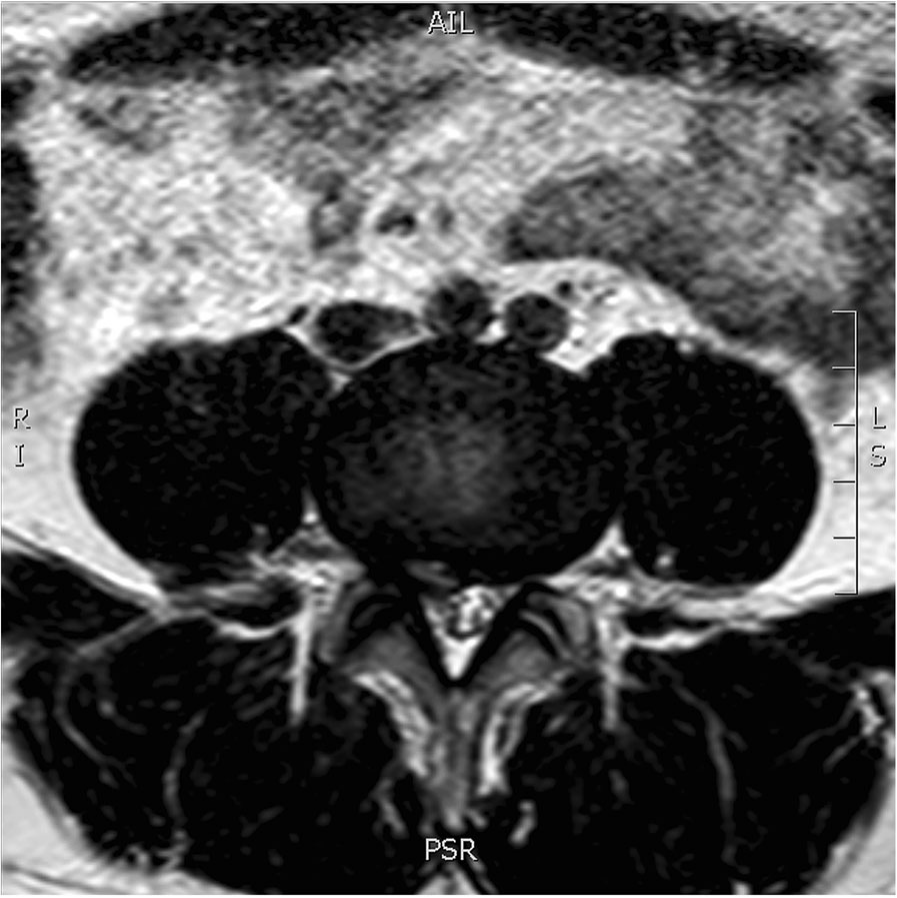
Axial T2-weighted MRI slice of a patient with LDH. LDH classification: MSU-2 (size) and right B (location); left FJOA: grade 0 (G0), and right FJOA: grade 2 (G2)
